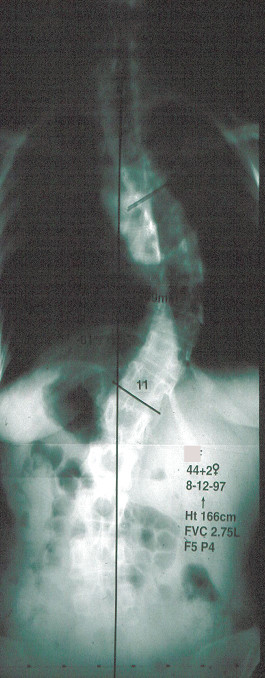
Posterior anterior radiographs of the same person as in Figure 1 at age 44 years, now with right thoracic idiopathic scoliosis of 61°. Her forced vital capacity (FVC) was 2.75 liters. Her function was normal and pain mild. (Reprinted with permission from Asher M, Burton DC: Natürlicher verlauf und langzeitauswirkungen der idiopathischen adoleszentenskoliose. In *Wirbel Säulen Deformitäten: Konservatives Management*. Edited by Weiss HR. München: Pflaum; 2003:97–107.)
